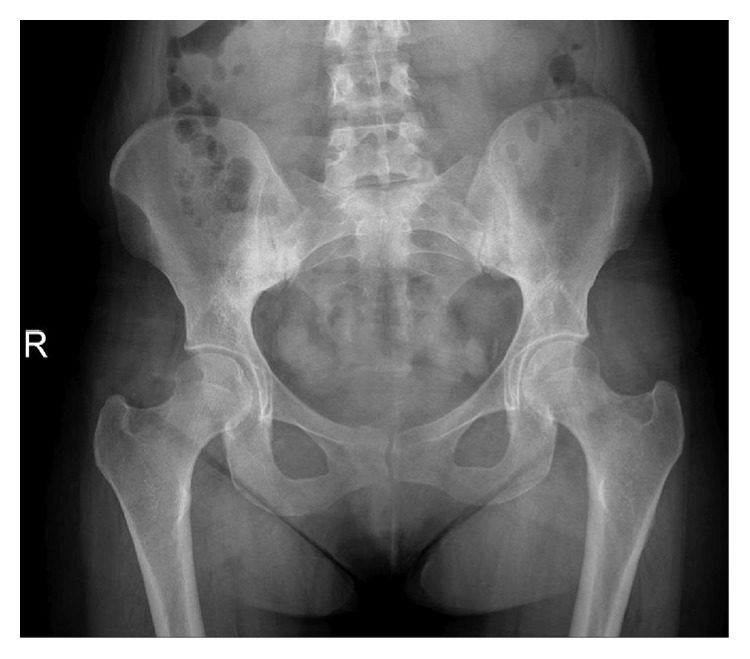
Plain radiograph of the sacroiliac joints demonstrating right grade 3 and left grade 2 sacroiliitis.
